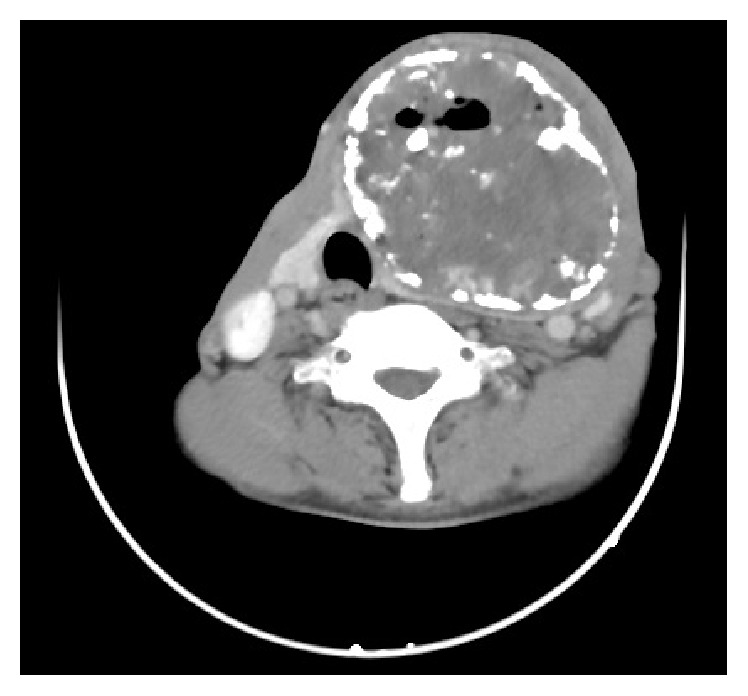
Computed tomography revealing enlarged left thyroid lobe with heterogeneous enhancement and calcifications. There was a hypodense component with focal air loculi within the lesion.
